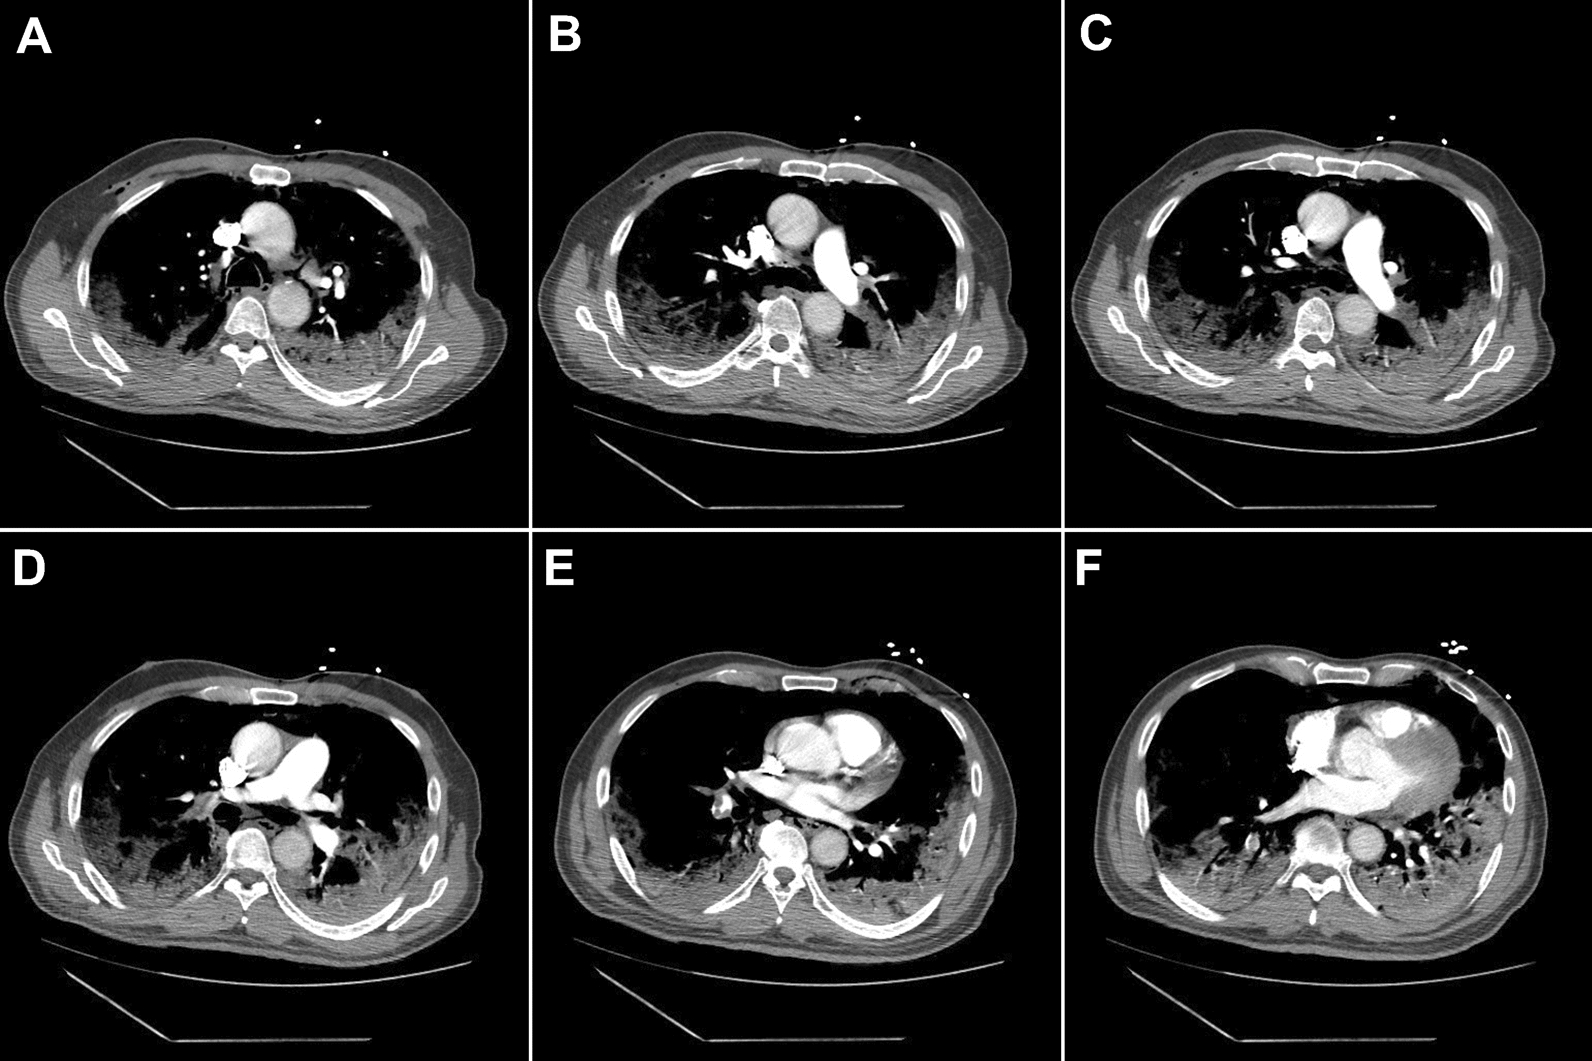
Computed tomography angiography of the chest obtained on hospital day 10. Through (A–F), there are proximal and peripheral filling defects in pulmonary arteries supplying all three lung fields on the right and peripheral branches on the left. There was no evidence of right heart strain. Significant bilateral pneumonia and pneumomediastinum were better visualized in the lung window shown in Fig. 3
